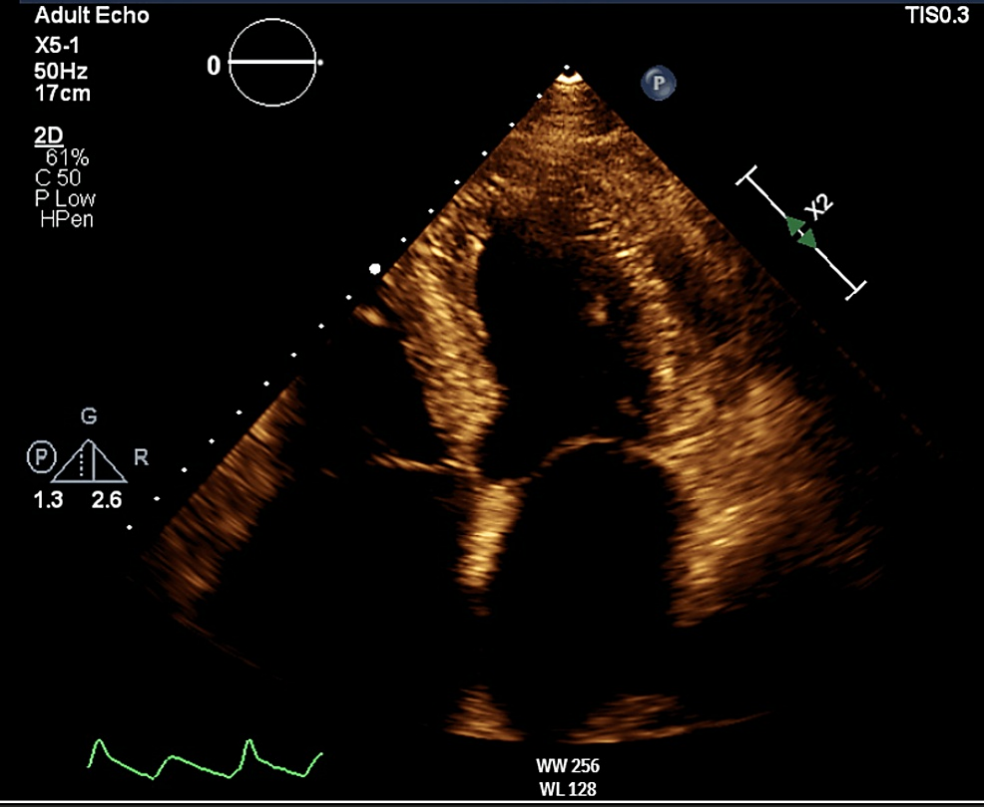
Two-dimensional four-chamber transthoracic echocardiogram (TTE) showing granular speckling, left ventricular hypertrophy, and severe biatrial dilatation.
